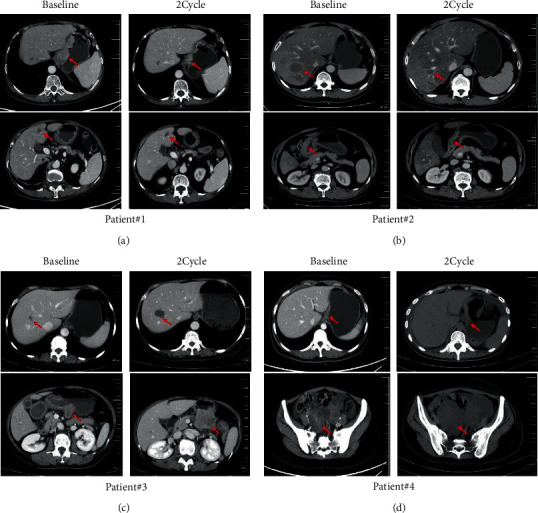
The clinical efficacy of drugs used in the patients based on the drug sensitivity results of the MiniPDX model. The red arrow indicates the location of the target lesion. CT scans were performed at the baseline and subsequent treatment cycles. Radiographic evidence of the four patients who achieved PR (patient #1, patient #2) or PD (patient #3, patient #4) after treatment was shown.
